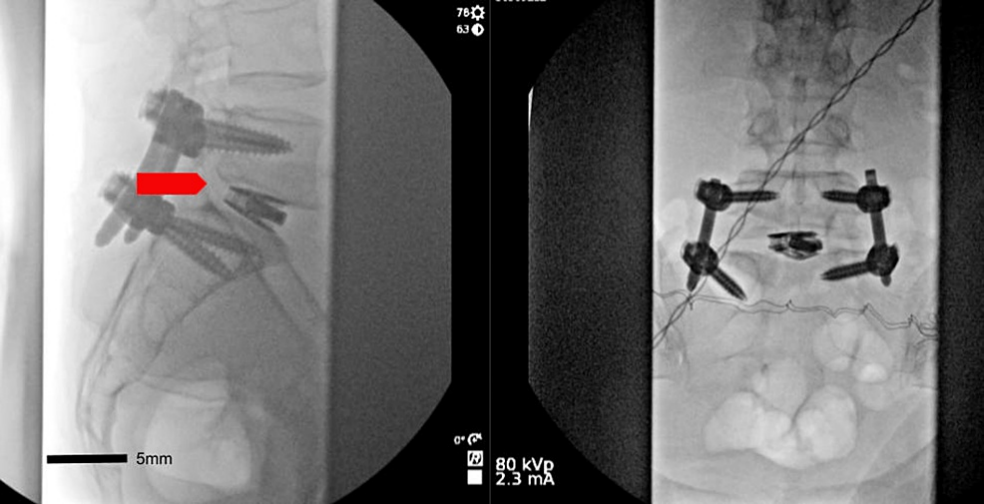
A lateral (left) and anterior/posterior (right) lateral plain film radiograph of the lumbar spine after the placement of the L5-S1 interbody graft and percutaneous pedicle screw fixation, with reduction of the spondylolisthesis (red arrow) seen on the lateral film
